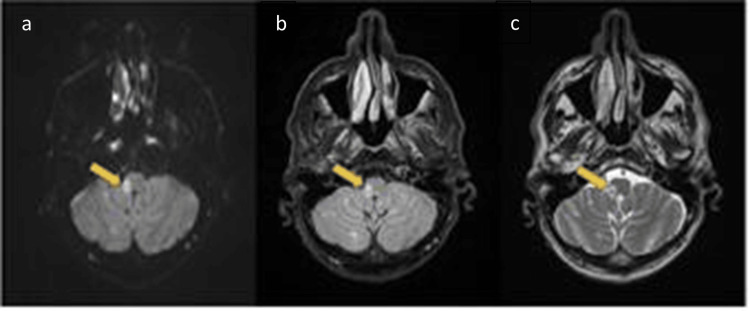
Brain magnetic resonance imaging showed signs of proximal intracranial right vertebral artery dissection with approximately 50% luminal reduction visible. Diffusion-weighted (a), fluid-attenuated inversion recovery (b), and T2-weighted (c) imaging sequences.
